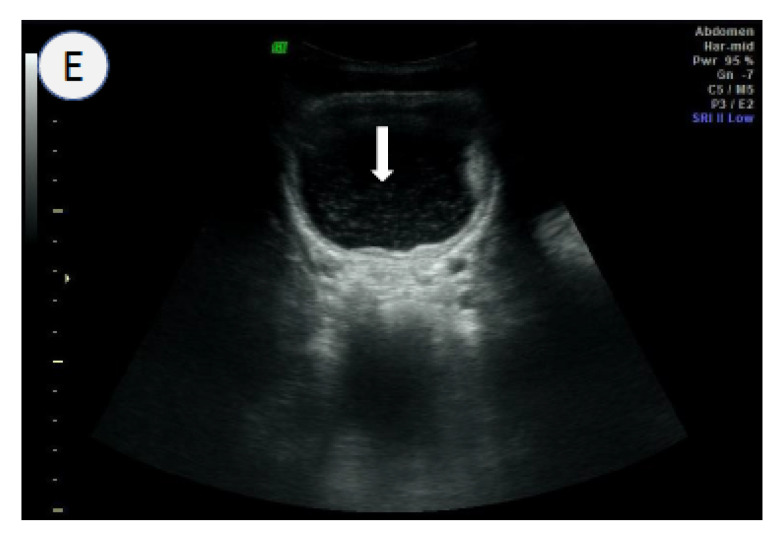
Ultrasound images of the urinary bladder in *S. haematobium* egg-positive Sudanese with the echogenic snow sign (arrows), wall thickening, and/or mass: (A) 14-year-old boy; (B) 18-year-old girl; (C) 20-year-old man; (D) 6-year-old girl; (E) 5-year-old-girl.
